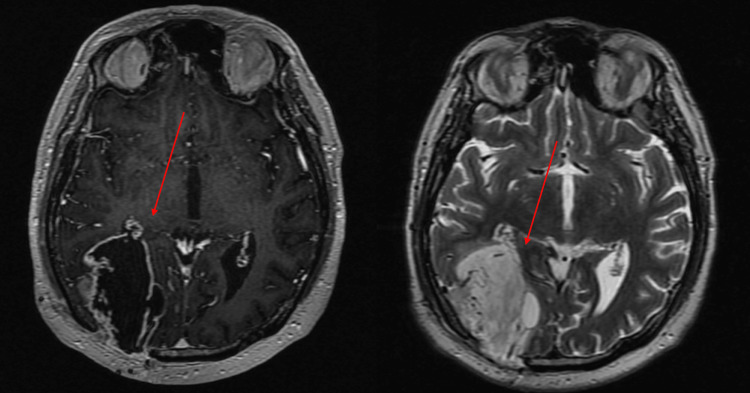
A. Cranial MRI in contrast-enhanced T1-weighted sequence with the presence of the right parietooccipital lesion with a halo uptake of the contrast medium. B. Cranial MRI in T2 sequence with evidence of the lesion with a cystic portion, well delimited, without the presence of peritumoral edema.
